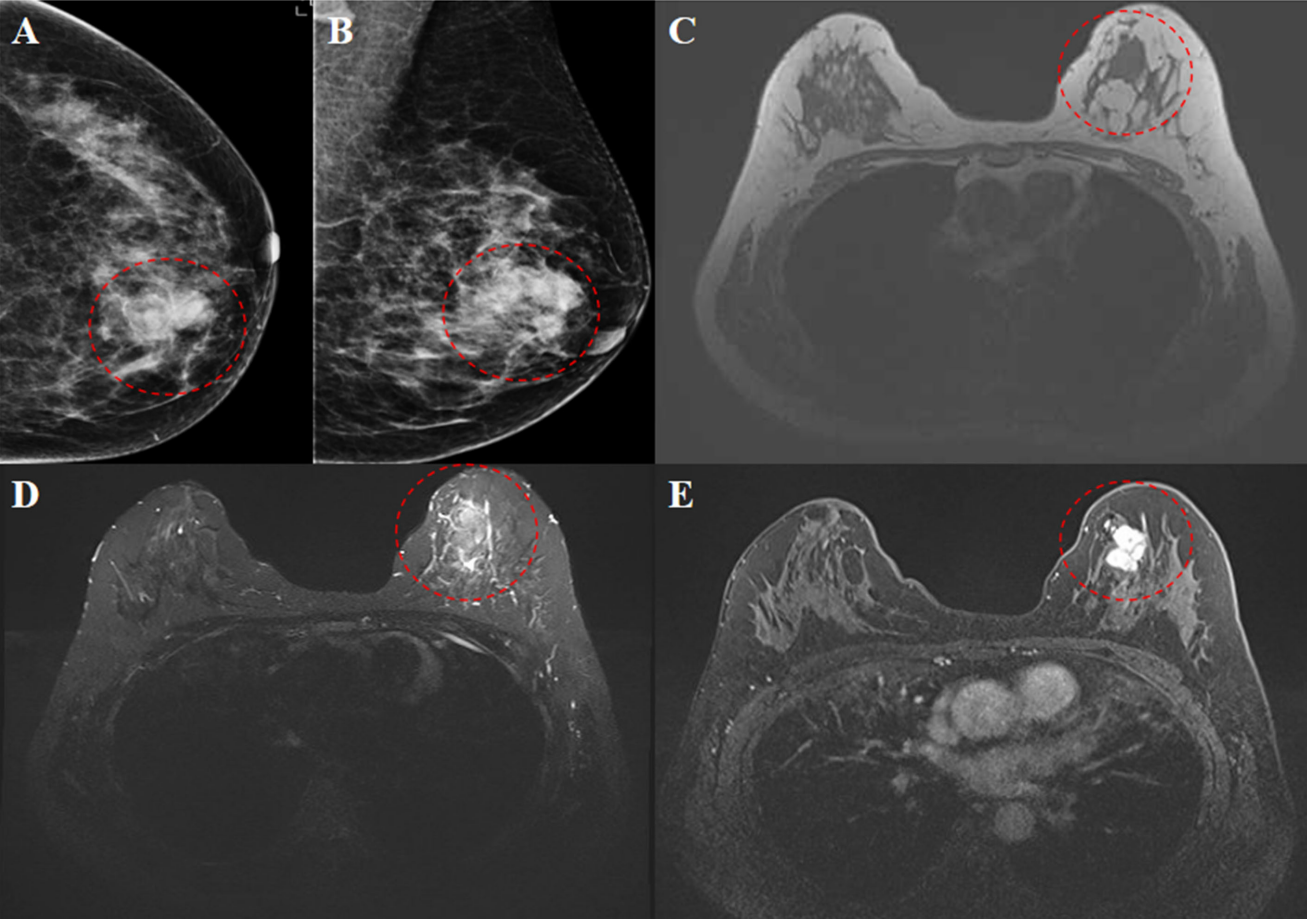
Mammography. High-density mass was identified in the upper inner quadrant of the left breast, characterized by indistinct margins and an irregular shape (A, B). MRI findings revealed low signal intensity on T1-weighted images (C) and slightly high signal intensity on T2-weighted images (D). Contrast-enhanced scans demonstrated uneven enhancement and segmented margins (E) (indicated by a red dashed circle).
